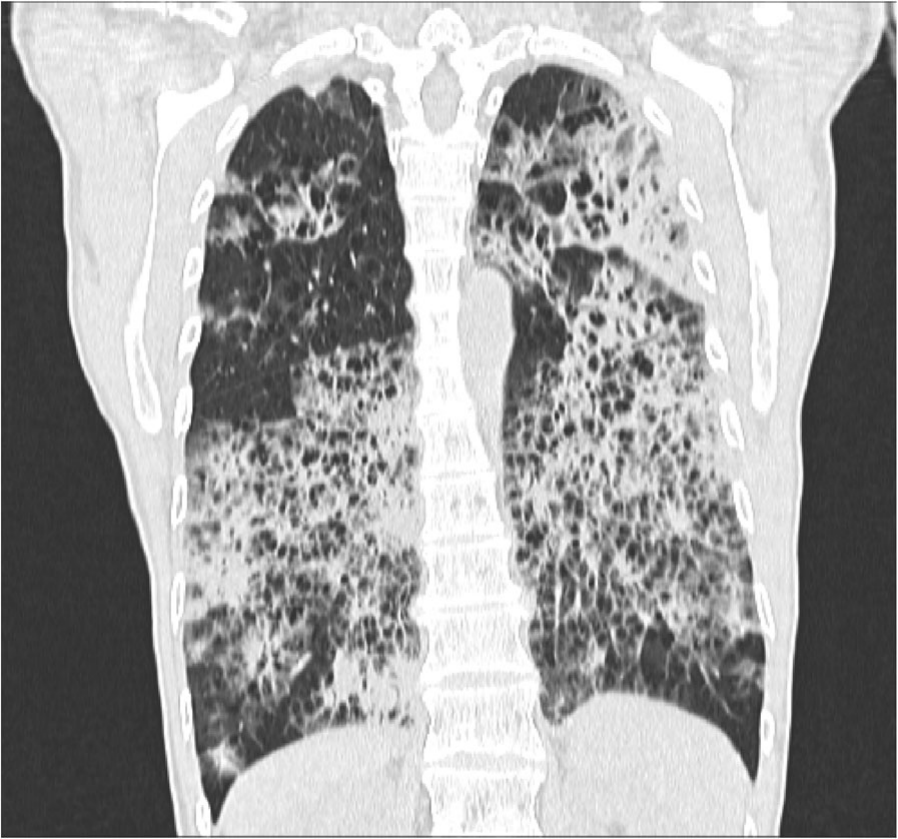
Computed tomography scan of the thorax with coronal view demonstrating of reticular interstitial opacities
